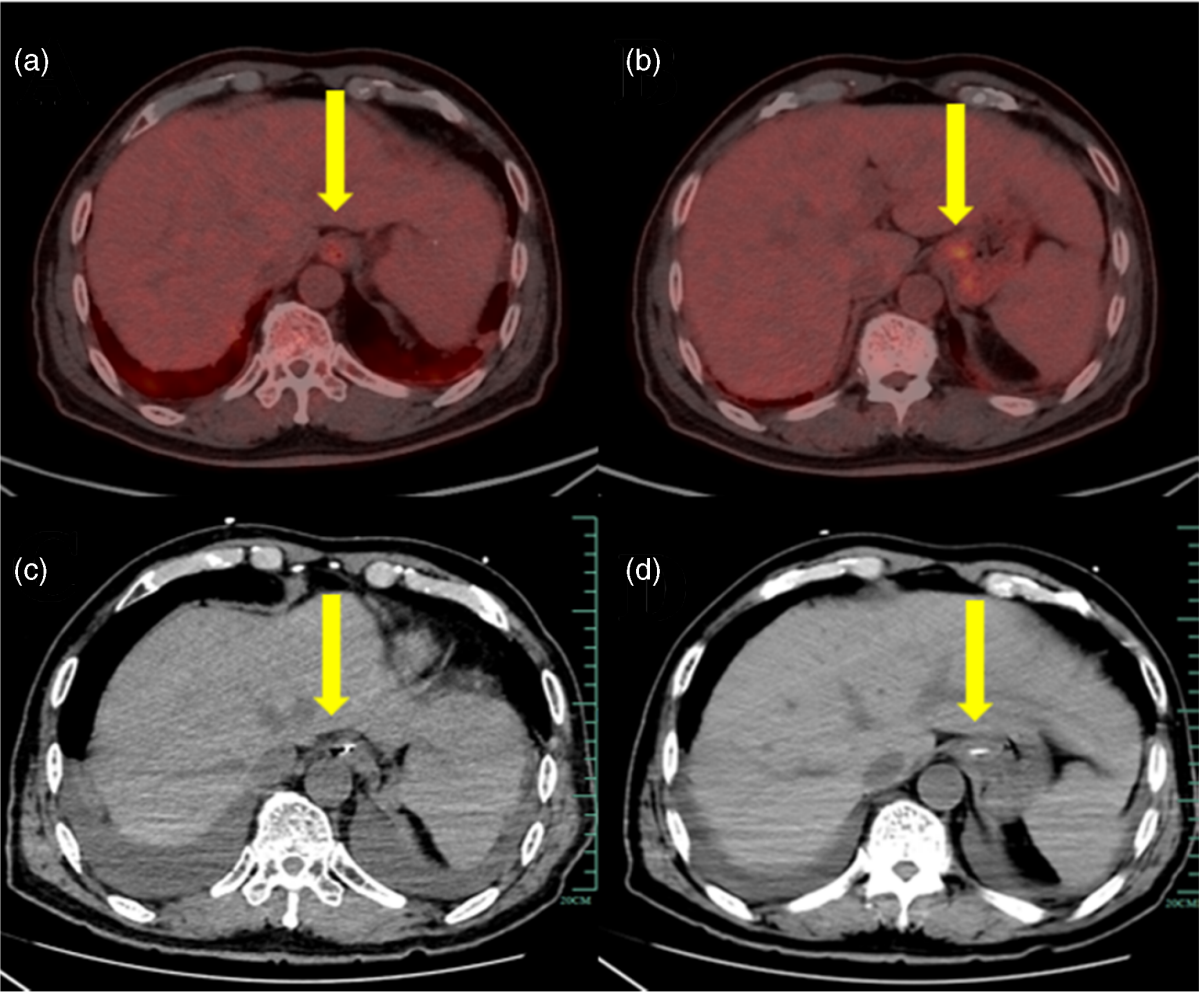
(a, b) Positron emission tomography‐computed tomography images showing increased fluorodeoxyglucose metabolism around the cardia, suggestive of inflammatory lesions. (c, d) Abdominal computed tomography images indicating the indwelling of the gastric tube at the cardia in the digestive tract lumen, with mucosal discontinuity.
